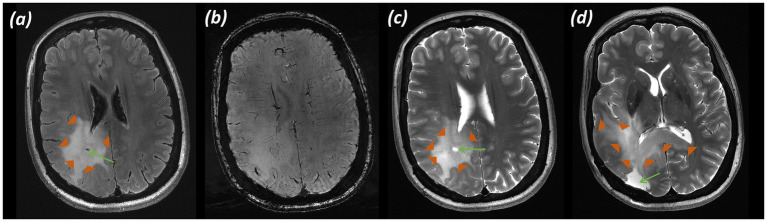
Patient 4: Glioblastoma, IDH-wildtype (CNS WHO grade 4). (a) T_2_*-*FLAIR image, (b) SWI image, and (c,d) T_2_-weighted TSE images at different slice levels through tumor and biopsy cavities. Orange arrowheads delineate tumor; green arrows mark resection cavities.
